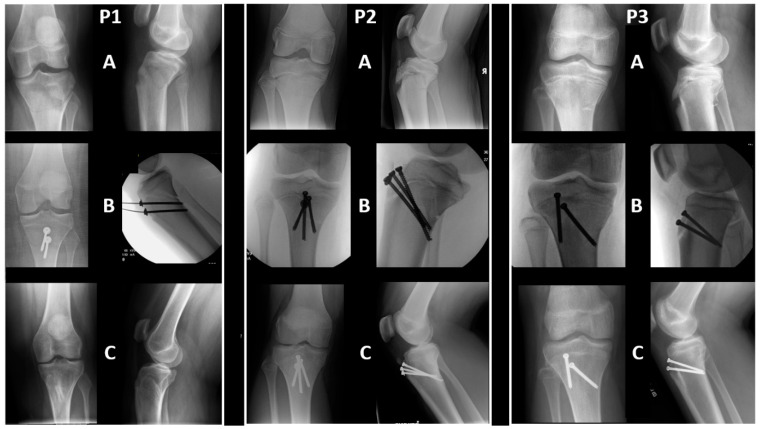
Salter–Harris II tibial tuberosity avulsion fractures (TTAF), treated surgically with a Distal Cortical Fixation (DCF). Salter–Harris II TTAF stands for a modified Watson–Jones type 4 TTAF fracture that propagates through the entire proximal tibial physis, starting at the anterior cortex and progressing all the way to the posterior cortex, with a posterior Thurston–Holland component of various sizes. A DCF is a fixation that captures the proximal fragment at its anterior cortex and captures the distal fragment at its posterior cortex. P1, P2, and P3 were three different patients. (A–C): Pre, intra (or immediately after), and final follow-up X-ray images, respectively.
